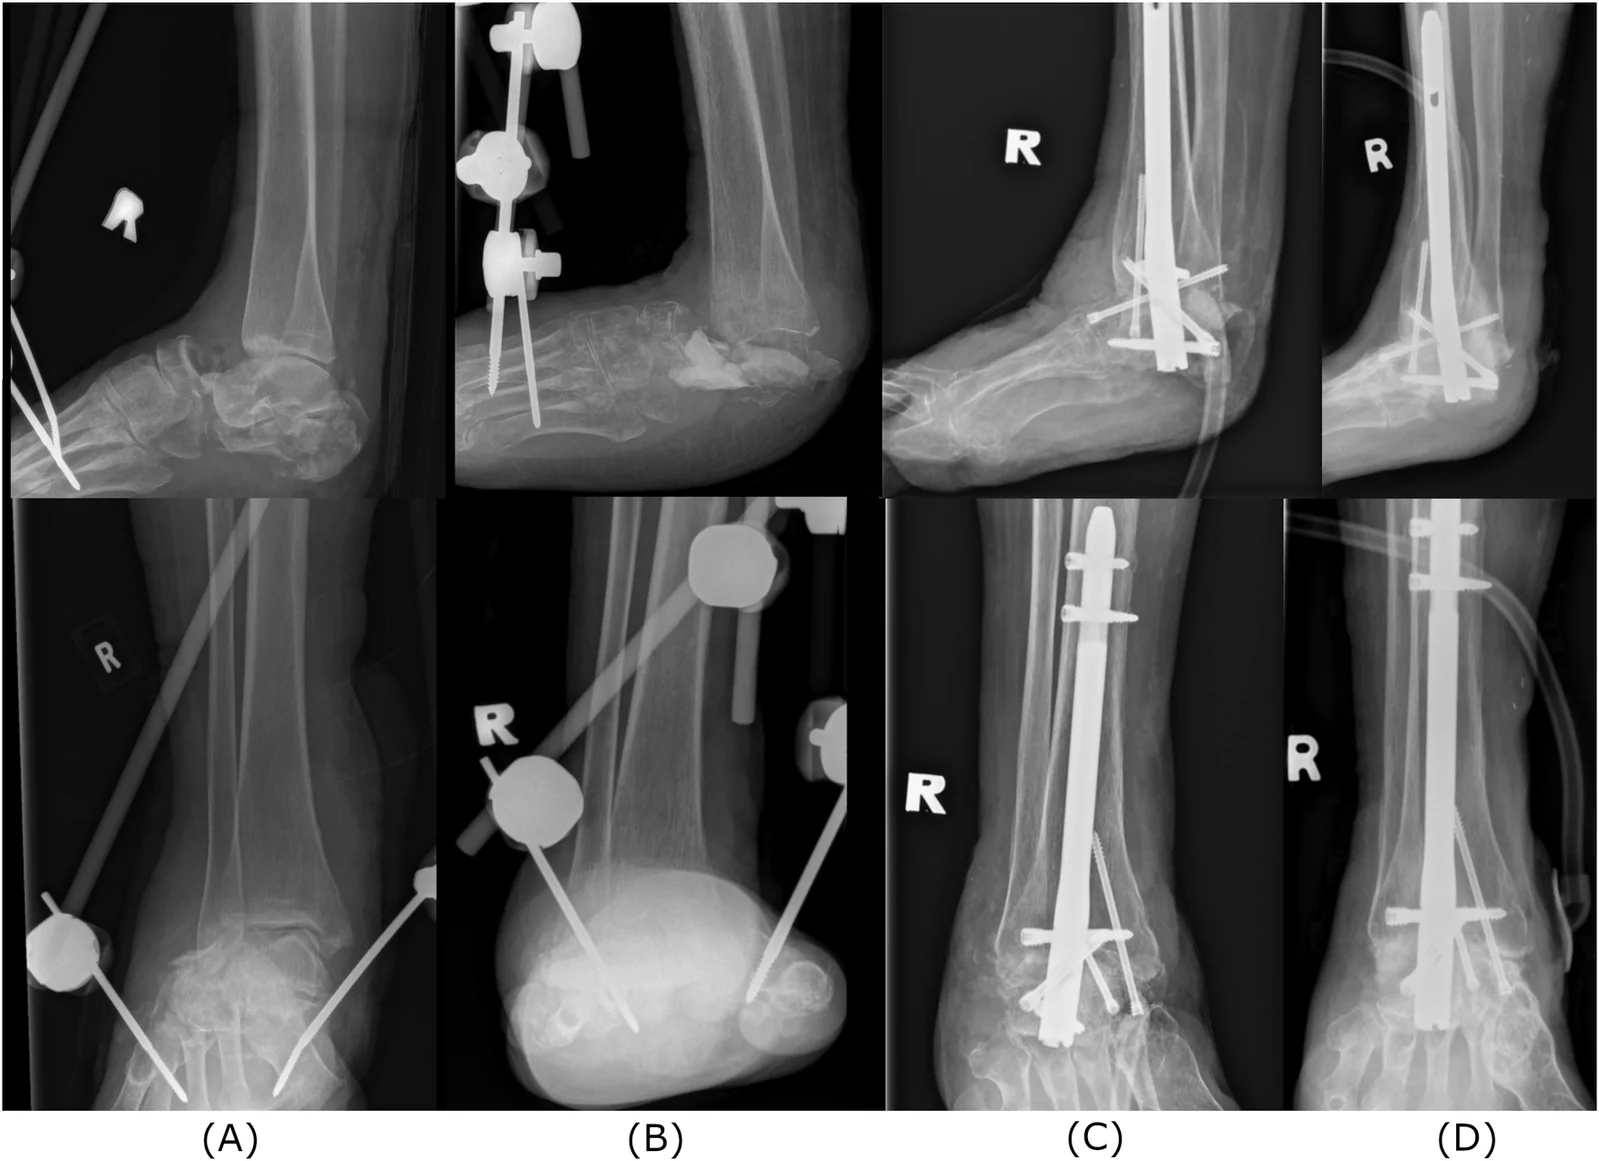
Staged radiographic documentation. (A) Initial injury with cross-ankle-joint external fixation. (B) Antibiotic-impregnated cement spacer *in situ* following partial talectomy and total calcanectomy. (C) Definitive hindfoot arthrodesis with structural femoral head allograft and a retrograde tibiotalocalcaneal arthrodesis nail. (D) Follow-up radiograph at 6 months.
